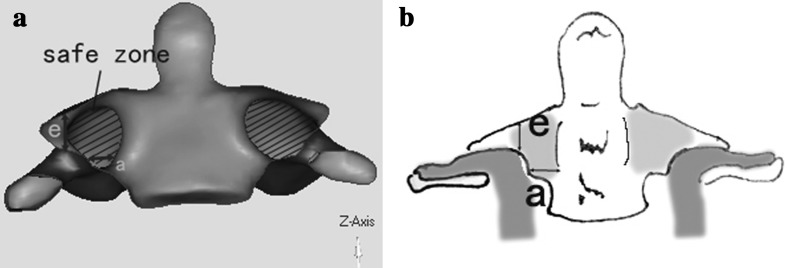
a reconstructed image of C2 CT scan showing a safe zone for pedicle screw placement boarded by parameter *a* and *e*. b VAG model figure could be reconstructed easily based on parameter *a* and parameter *e* measured on the consecutive CT scan of C2
